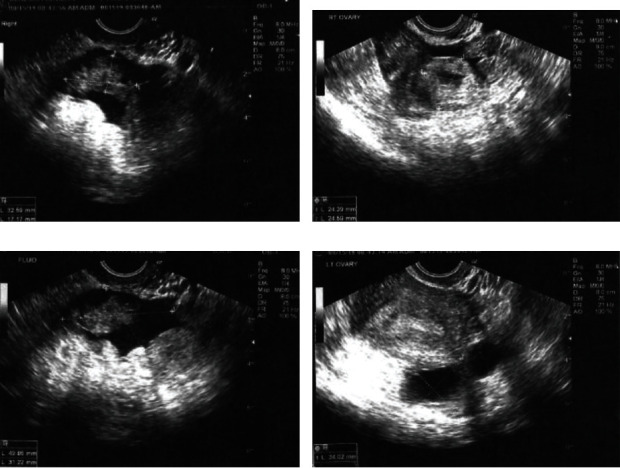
Transvaginal ultrasound findings. (a) Right adnexa. (b) Right ovary. (c) Pelvic fluid. (d) Left ovary.
